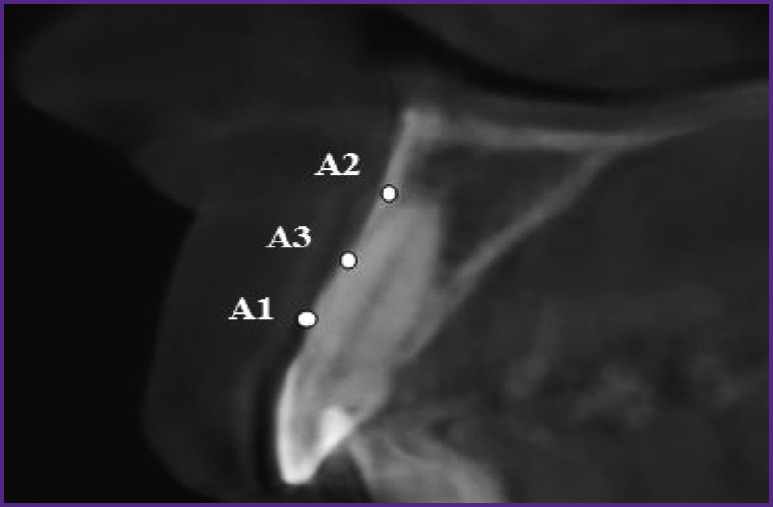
Measuring the facial alveolar bone wall thickness in the region of tooth 1.1 using cone-beam CT (mm)
